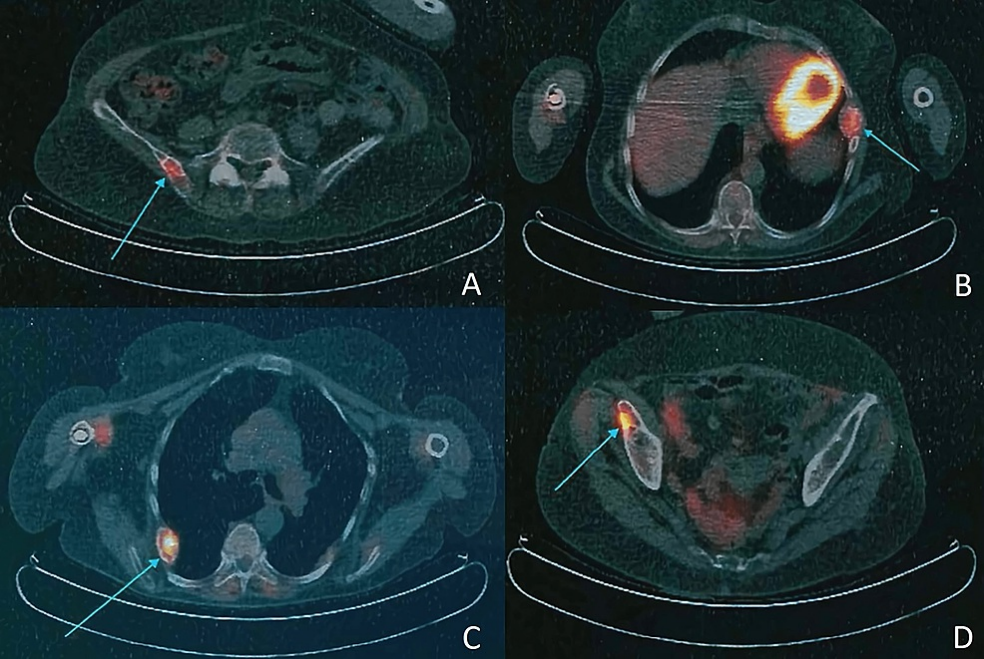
F-18-FDG positron emission tomography showing high focal uptake on the right iliac wing (A), rib cage (B, C), and right acetabulum (D).
